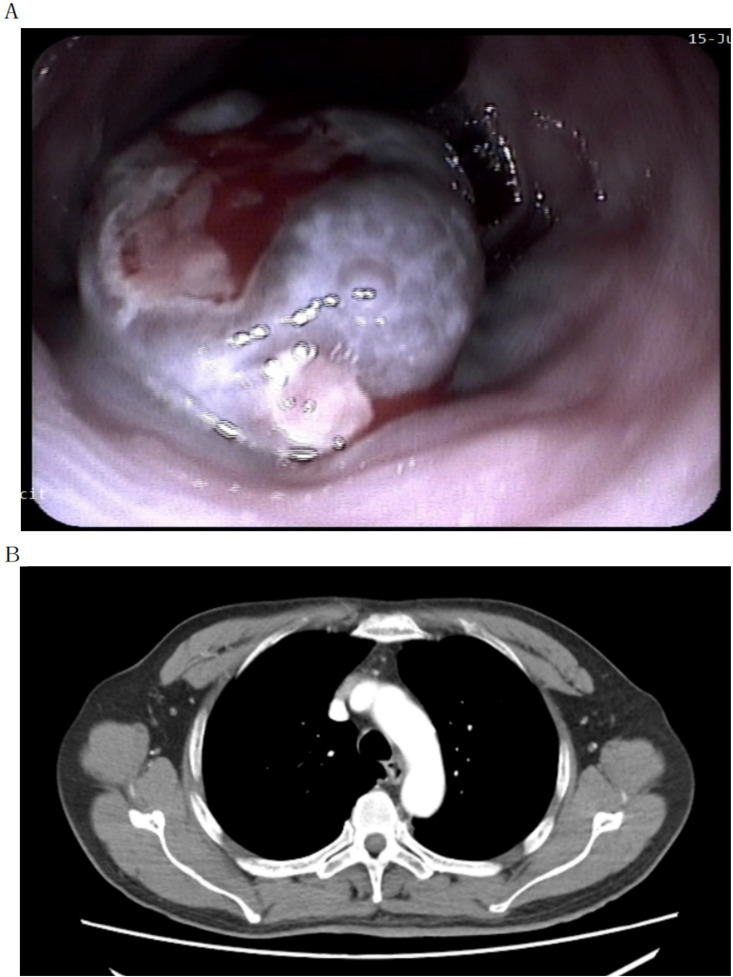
Diagnostic imaging findings. (A) Endoscopy and Endoscopic Ultrasound (EUS) revealing a longitudinal, blue-colored, polypoid mass (approx. 2.0 cm × 4.0 cm) located 27 cm from the incisors. (B) Contrast-enhanced CT of the chest showing thickening and enhancement of the middle esophageal wall without obvious mediastinal lymphadenopathy.
